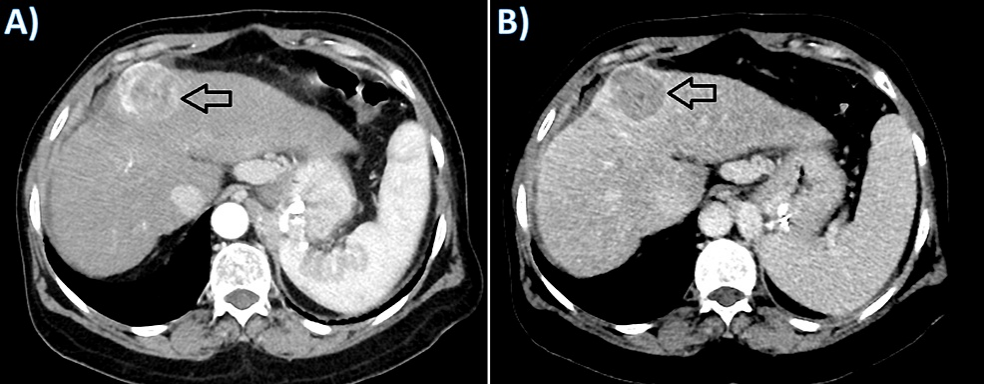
Axial contrast enhanced CT scan images. A: arterial phase and B: portovenous phase showing cirrhotic liver with hypervascular partially exophytic lesion in segment IV measuring 3.8 cm x 4 cm suggestive of HCC. HCC, hepatocellular carcinoma
